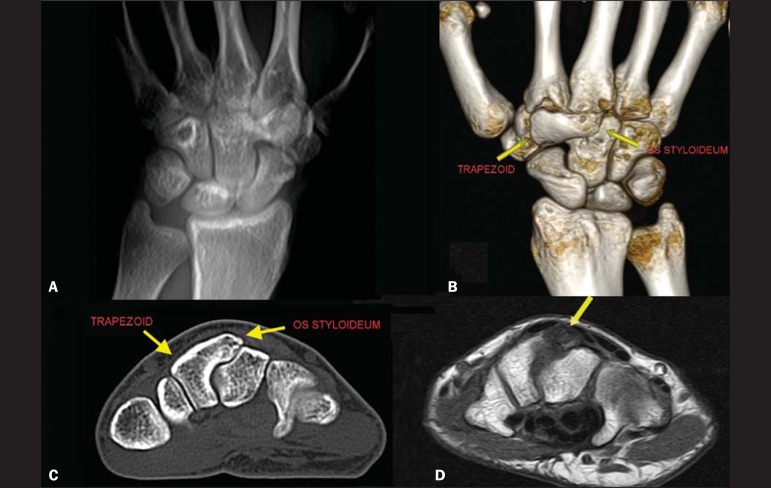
A: Digital X-ray showing a lack of definition of the joint space
